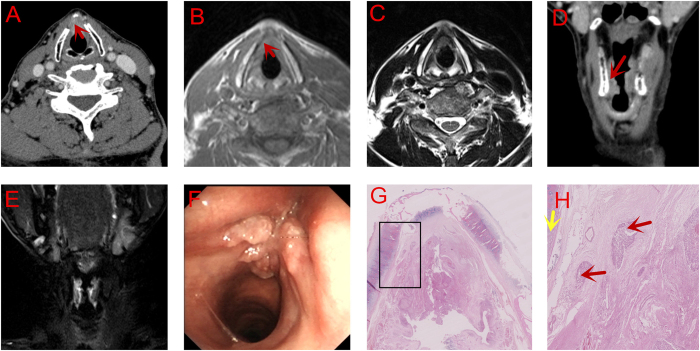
PGS Invasion without Thyroid Cartilage Involvement. (A) (Axial) and (D). (Coronal) CT scan: Involvement of thyroid cartilage (red arrow). (B) (Enhanced T1WI, Axial), (C). (T2WI, Axial) and E. (T2WI, Coronal) MRI scan: Without involvement of thyroid cartilage. (F) Electronic laryngoscopic examination. (G,H) (amplified image within the rectangle in G., 40 ×) Serial section: Without involvement of thyroid cartilage (Red arrow: tumor cells; Yellow arrow: thyroid cartilage).
